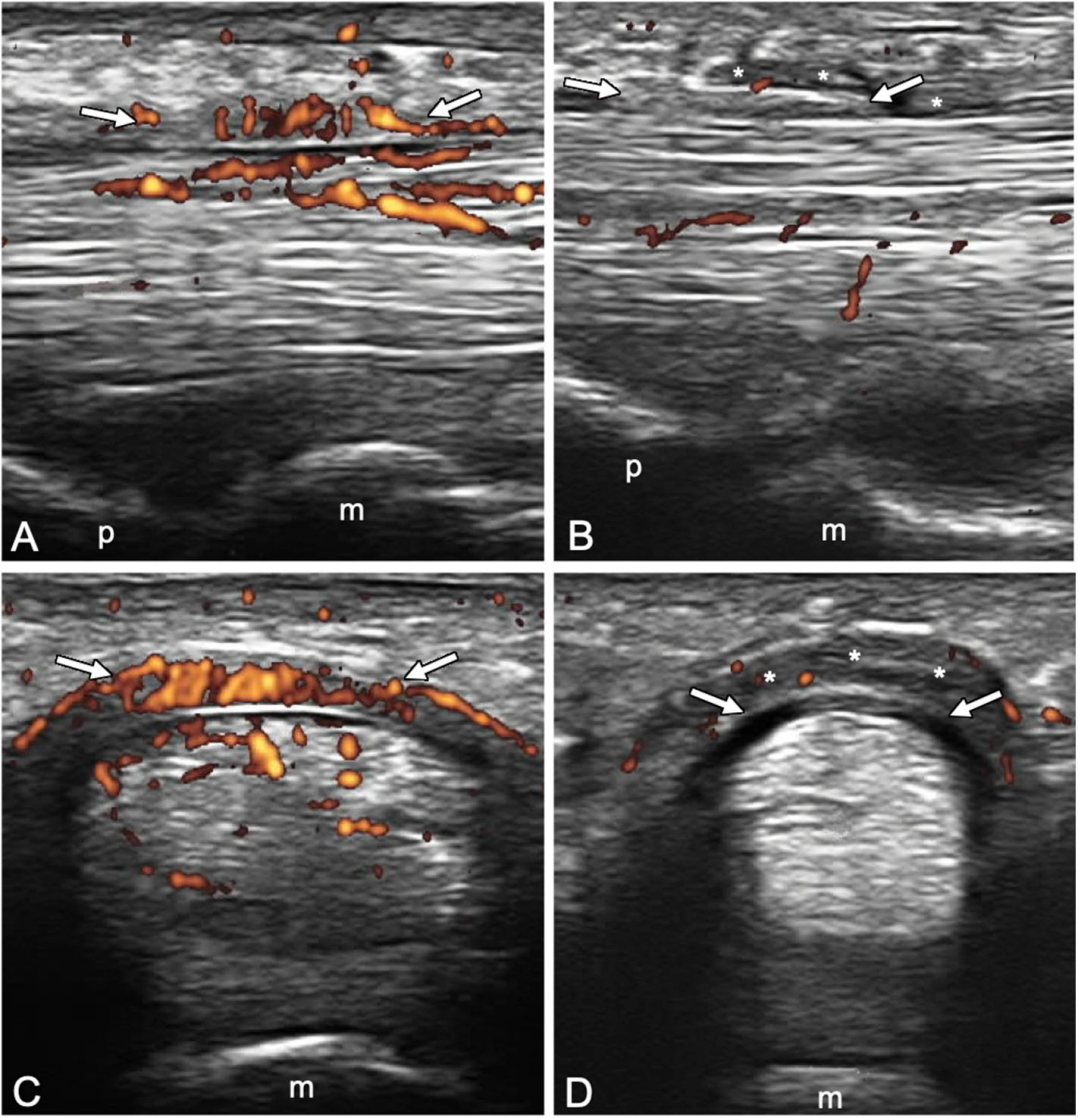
Longitudinal (A,B) and transverse (C,D) scans of the annular pulley A1 using a 22-MHz probe in psoriatic arthritis (A,C) and rheumatoid arthritis (B,D) patients. In (A,C), A1 pulley (arrows) inflammation is depicted (power Doppler signal inside a thickened pulley). Power Doppler signal is also noticeable within the superficial flexor tendon in the portion closest to the pulley. In (B,D), A1 pulley (arrows) is not inflamed. The presence of synovial proliferation in the tendon sheath spilling over the normal pulley is shown (asterisks), representing a potential diagnostic pitfall. m, metacarpal head; p, proximal phalanx.
